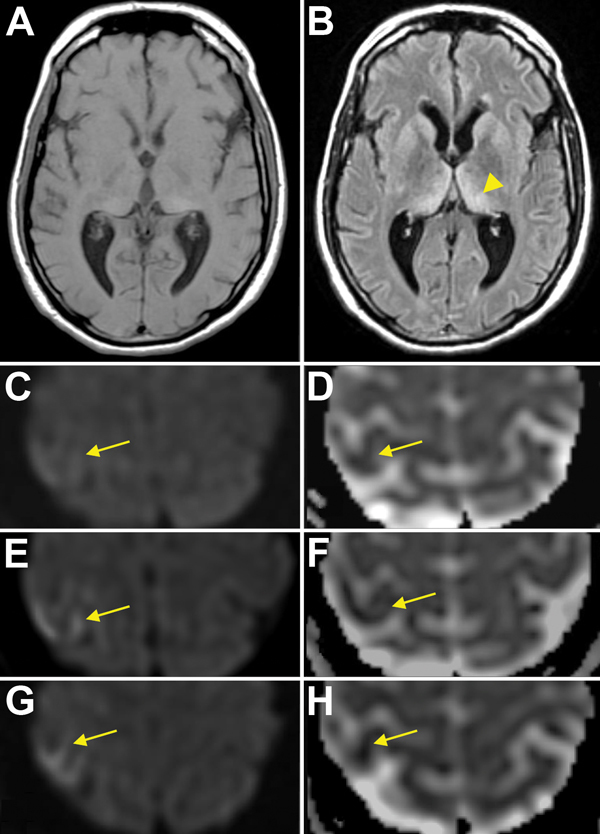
Magnetic resonance imaging (MRI) results for a US patient with variant Creutzfeldt-Jakob disease. T1 sequence (A) and T2 FLAIR sequence (B) show the “pulvinar” or “hockey stick” sign (arrowhead). Initial diffusion weighted imaging (DWI) (C) and apparent diffusion coefficient (ADC) (D) images show subtle restricted diffusion in the right primary motor cortex (arrows). Subsequent MRIs show increasing hyperintensity (arrows) on DWI (E, then G) and further attenuation (arrows) on ADC (F, then H), consistent with the “cortical ribbon” sign.
